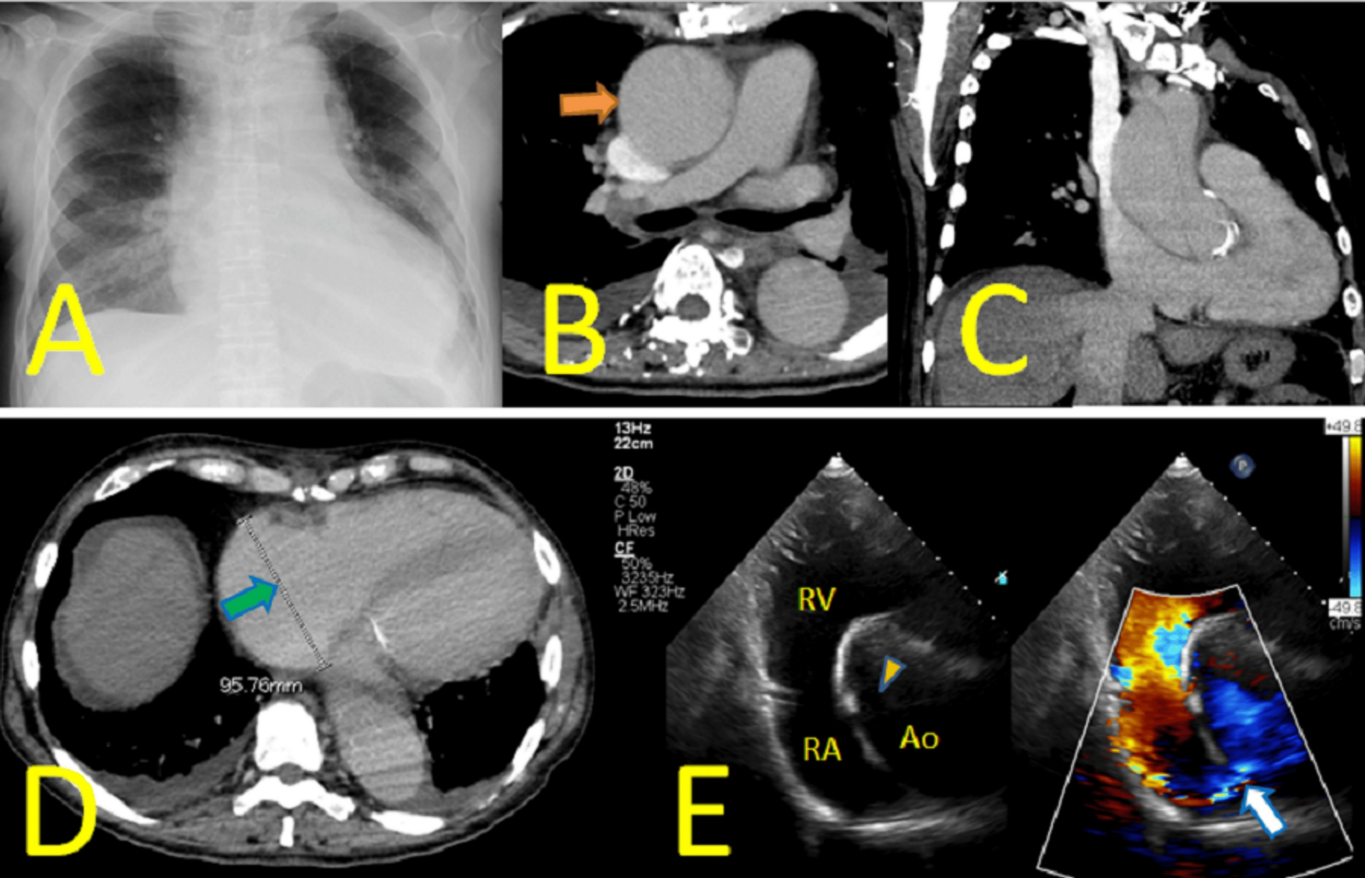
Preoperative findings of type A aortic dissection A: Chest radiography revealed cardiac and mediastinal enlargement. B: Contrast-enhanced CT showed that the dissected ascending aorta had enlarged to 60 mm in diameter (orange arrow). C: The reduced use of contrast agent made it difficult to identify the dissection entry point. D: The right atrium had enlarged to 96 mm (green arrow). E: Transthoracic echocardiography revealed a shunt flow (white arrow) from the false lumen of the ascending aorta (Ao) to right atrium (RA). The yellow arrowhead indicates the medial flap. RV is right ventricle.
